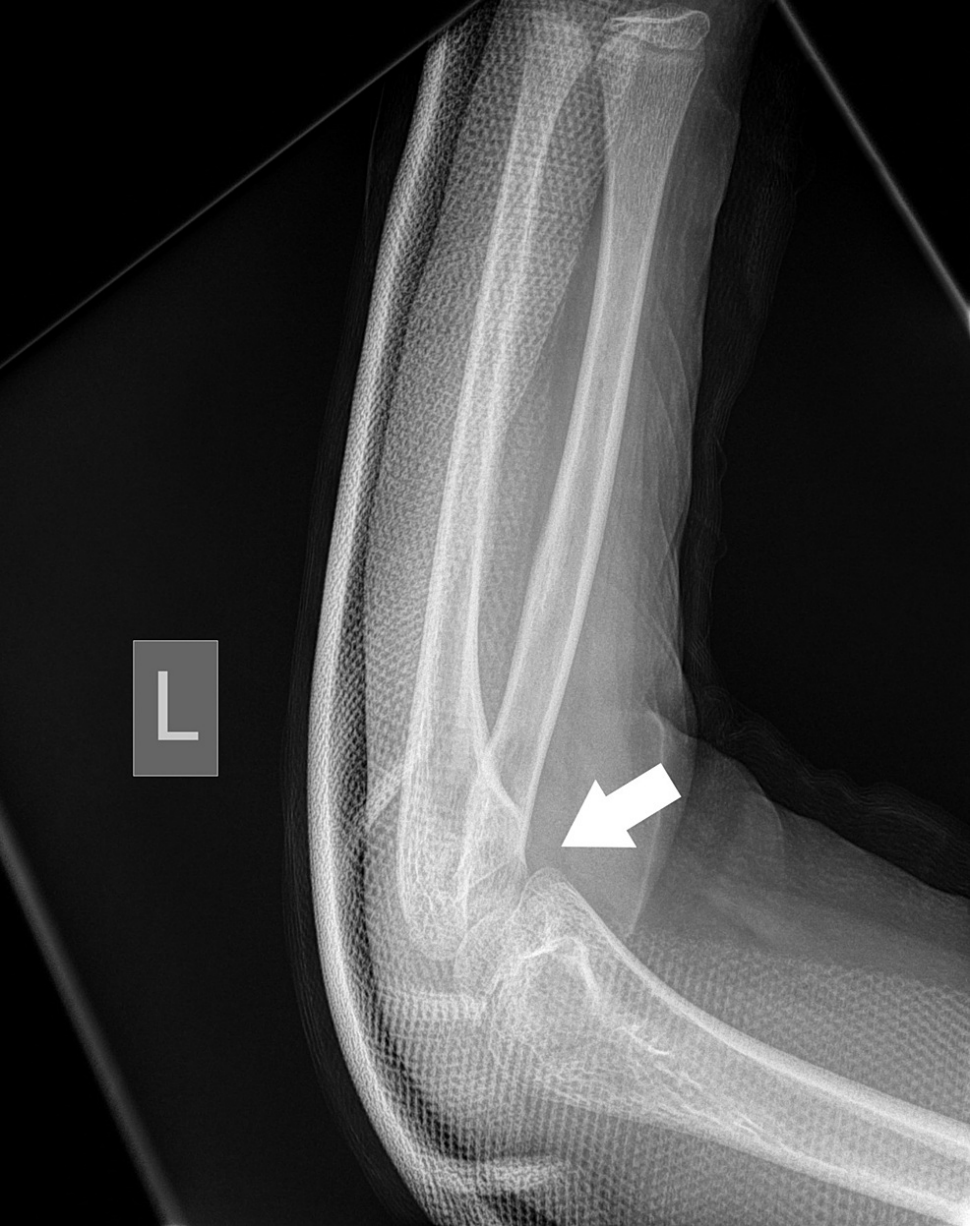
Postoperative x-ray of left (L) elbow after closed reduction (white arrow) (view 2).
